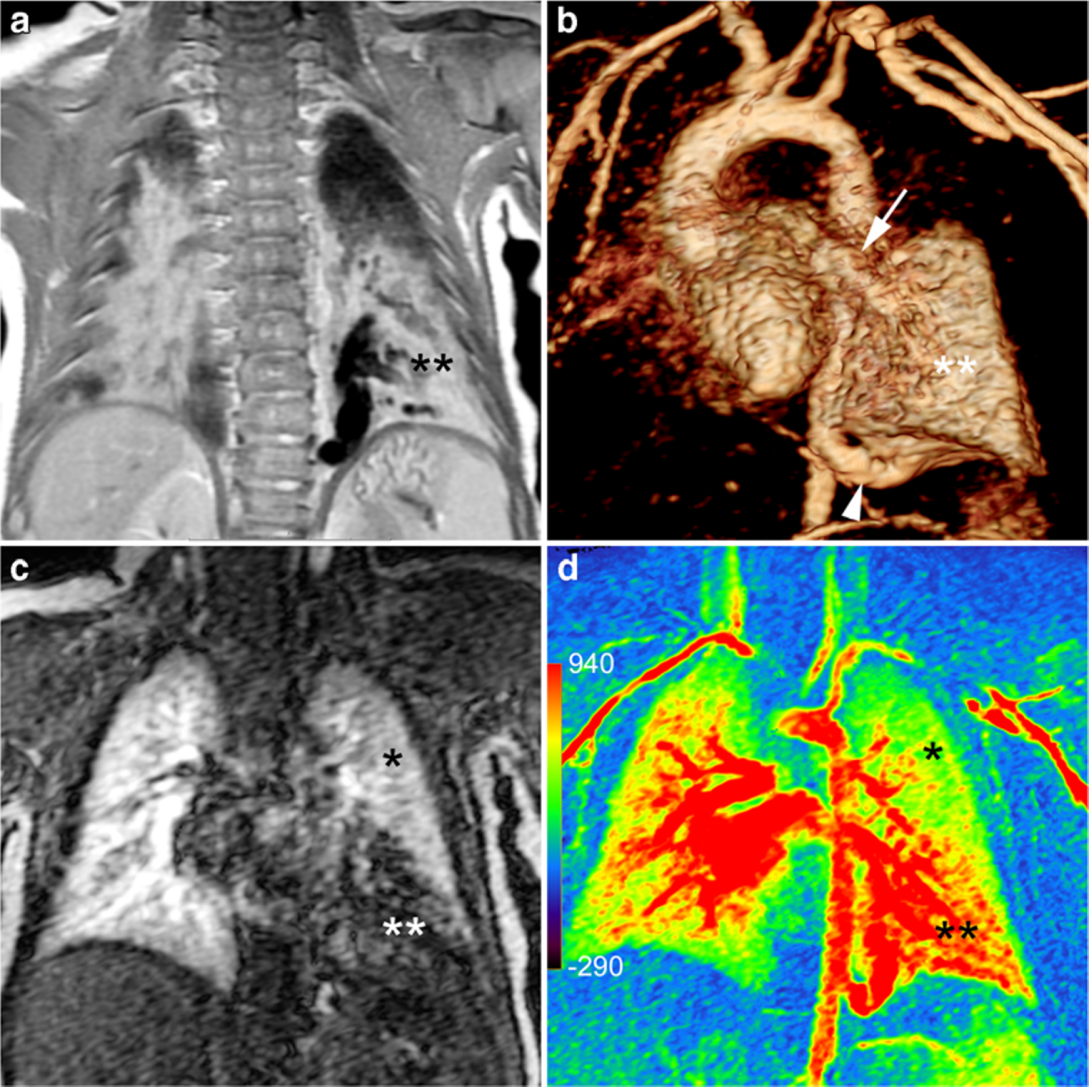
Bronchopulmonary sequestration in a 3-month-old boy. a Coronal proton density MR image shows consolidation in the left lower lobe () and prominent vessels with flow voids. b Left anterior oblique volume rendered angiographic view shows the lung lesion (), its systemic arterial supply (*arrowhead*) from the descending aorta and venous drainage (*arrow*) to the left lower pulmonary vein. c, d Coronal image at peak pulmonary enhancement (c) shows a perfusion defect () and parametric map (enhancement integral) (d) shows increased perfusion () compared to normal lung (* in c and d)
